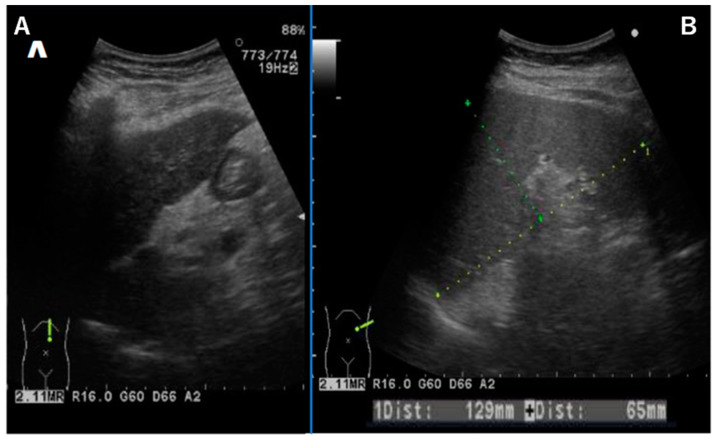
Abdominal ultrasound showing a liver contour with an irregular appearance (A). The spleen was enlarged, and ascites were absent (B).
